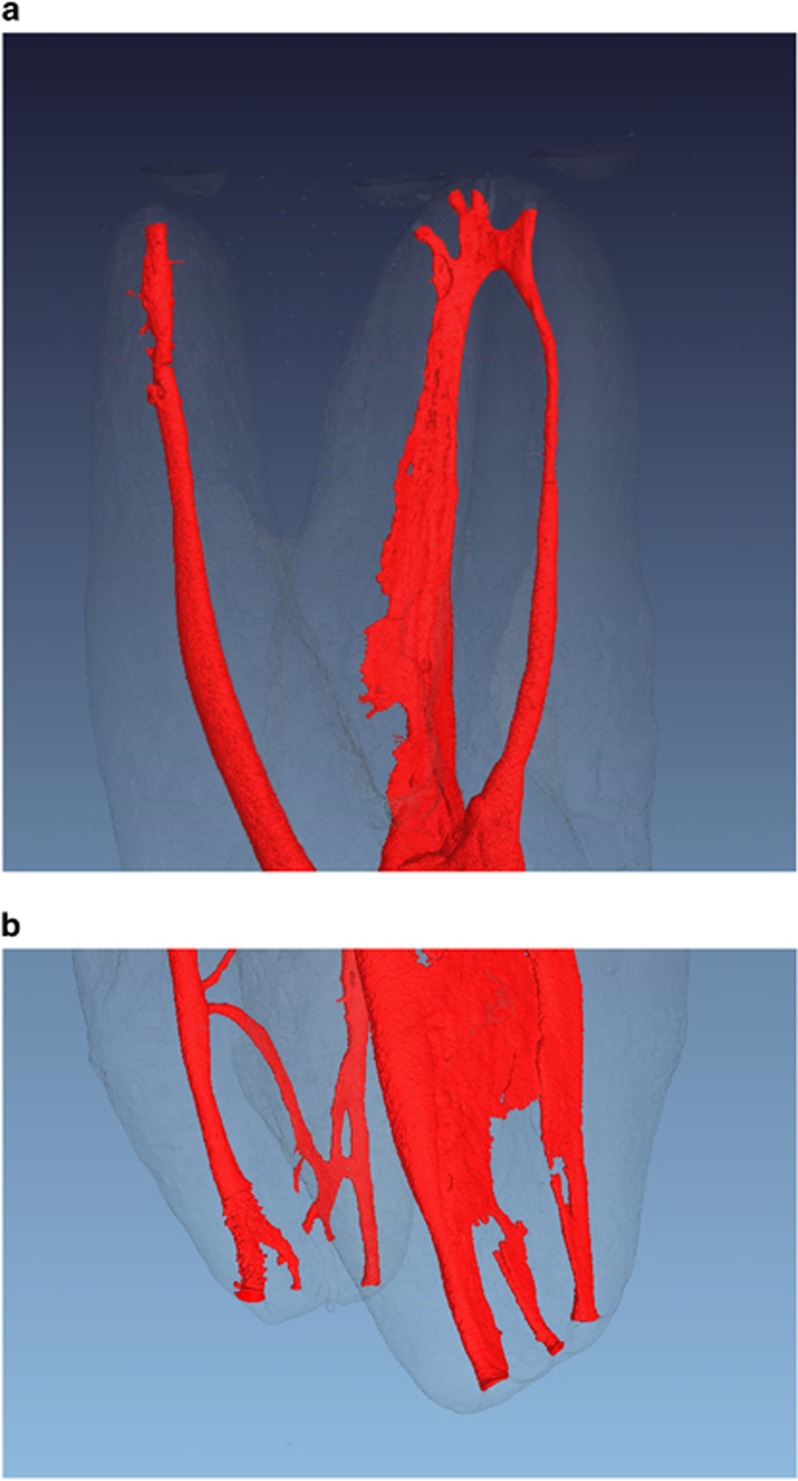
Micro-computed tomography of a maxillary (a) and mandibular (b) molar, respectively. It can be denoted the complexity of the root canal system morphology, specially of the apical area where the portals of communication with the periapical tissues clinically demand an accurate preparation an hermitic seal.
